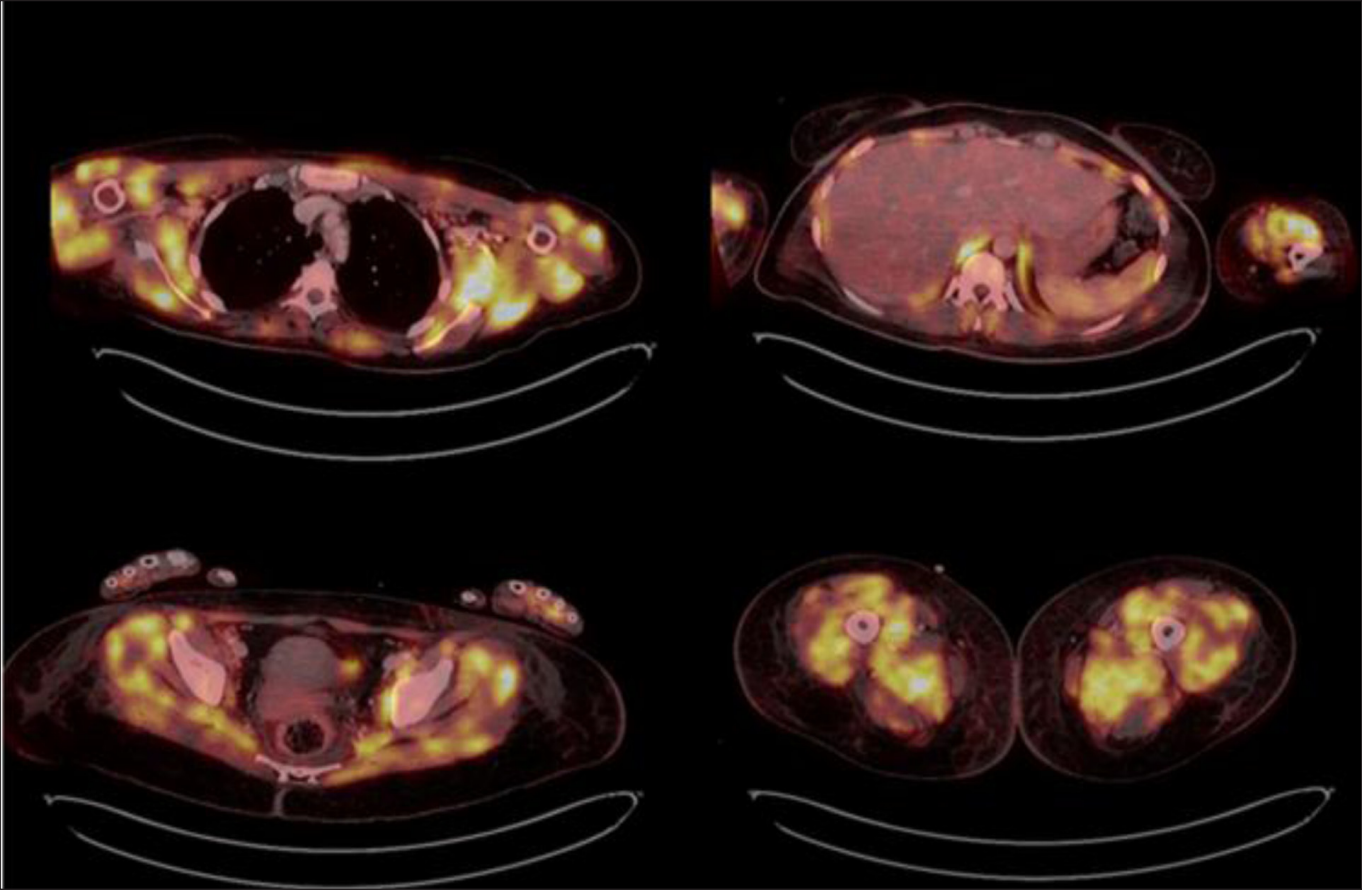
Diffuse FDG uptake in the shoulder, hip girdle muscles, as well as in the intercostal and lower limb muscles.
